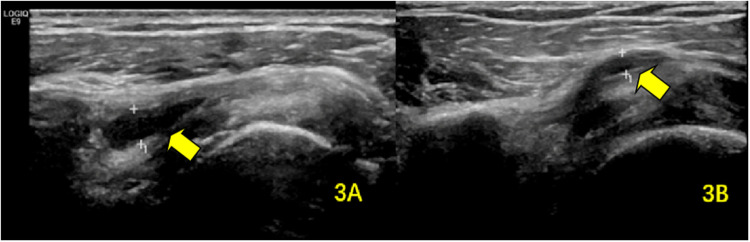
SA–SD bursal effusion demonstrated on ultrasonography (A) at baseline and (B) following rehabilitation. The arrows highlight the pathological fluid accumulation within the SA–SD bursa.
